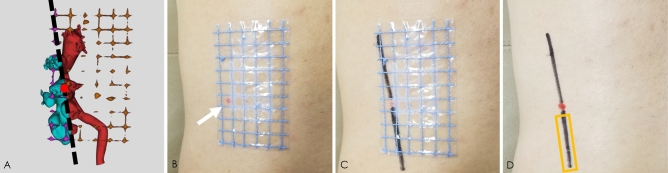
Location of the skin puncture point and ultrasonic probe. (A) Skin puncture point (red dot) and tangent of section C are located on the grid patch (black line segment). (B) Point of skin puncture (red spot indicated by the white arrow) was located on the skin of the patient using the grid surface patch. (C) Tangent of section C, which was restored on the skin of the patient using a grid surface patch (black line segment). (D) Tangent (black segment in the golden box) of section C on the grid surface patch, which is where the ultrasonic probe was placed.
